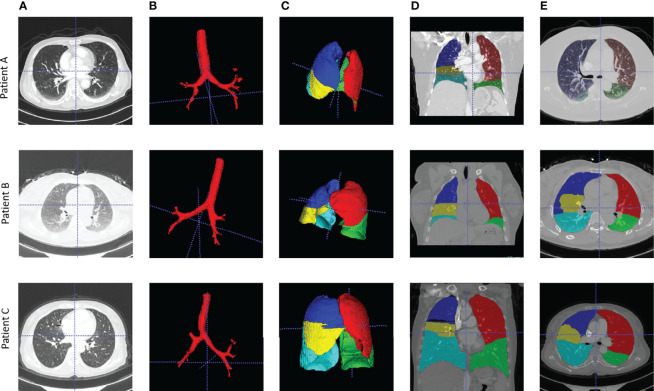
Examples of airway and lung lobes segmentation. (A) Patient raw CT scan; (B) Airway segments produced by region-growing algorithm; (C) lung lobes segments, 3D; (D) Lung lobes segments, x-axial 2D; (E) Lung lobes segments, z-axial 2D.
